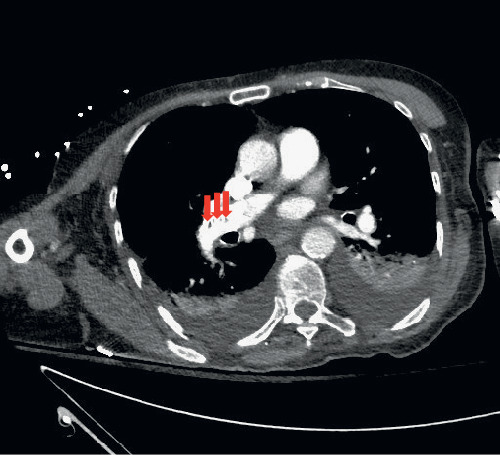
CT angiogram showing cement emboli (arrows) in the right lung.
